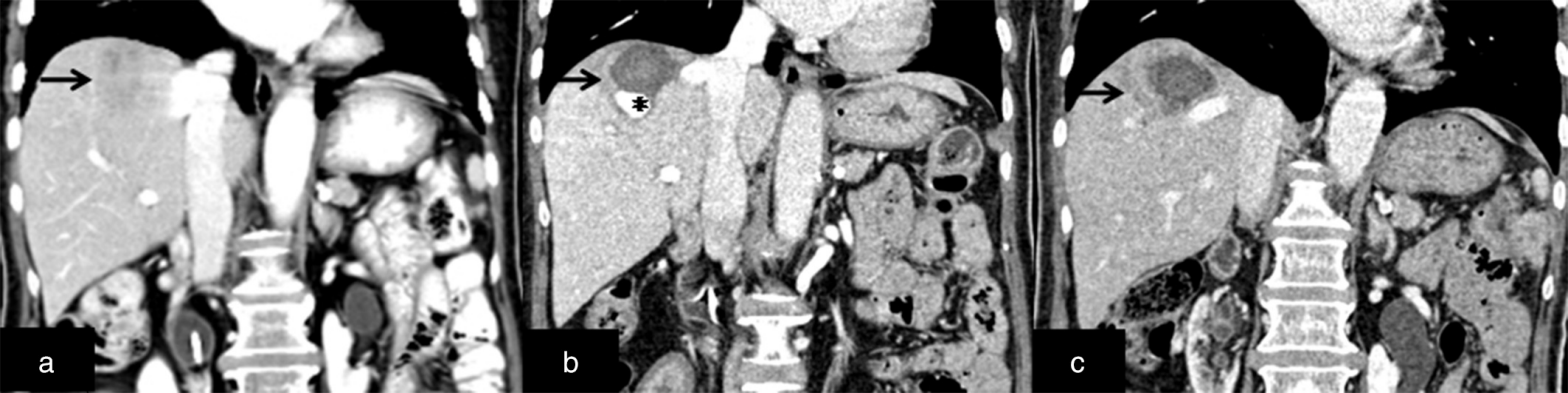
Contrast-enhanced CT scan of the abdomen in the portal venous phase performed at baseline (a), 6 weeks (b) and 12 weeks (c) after the ablation. CT scan at baseline (a) showed a heterogeneously enhancing hypodense metastatic lesion (arrow) in segment VIII. CT scan of the abdomen 6 weeks after ablation (b) revealed residual peripheral tumour with an intensely enhancing area (*) within, measuring 2.3 (width) × 1.4 (AP) cm and suggestive of a pseudoaneurysm. The pseudoaneurysm arises inferolaterally along the electrode pathway. There was resolution of the pseudoaneurysm with increased residual peripheral tumour (arrow) on the CT scan performed 12 weeks after the ablation (c).
